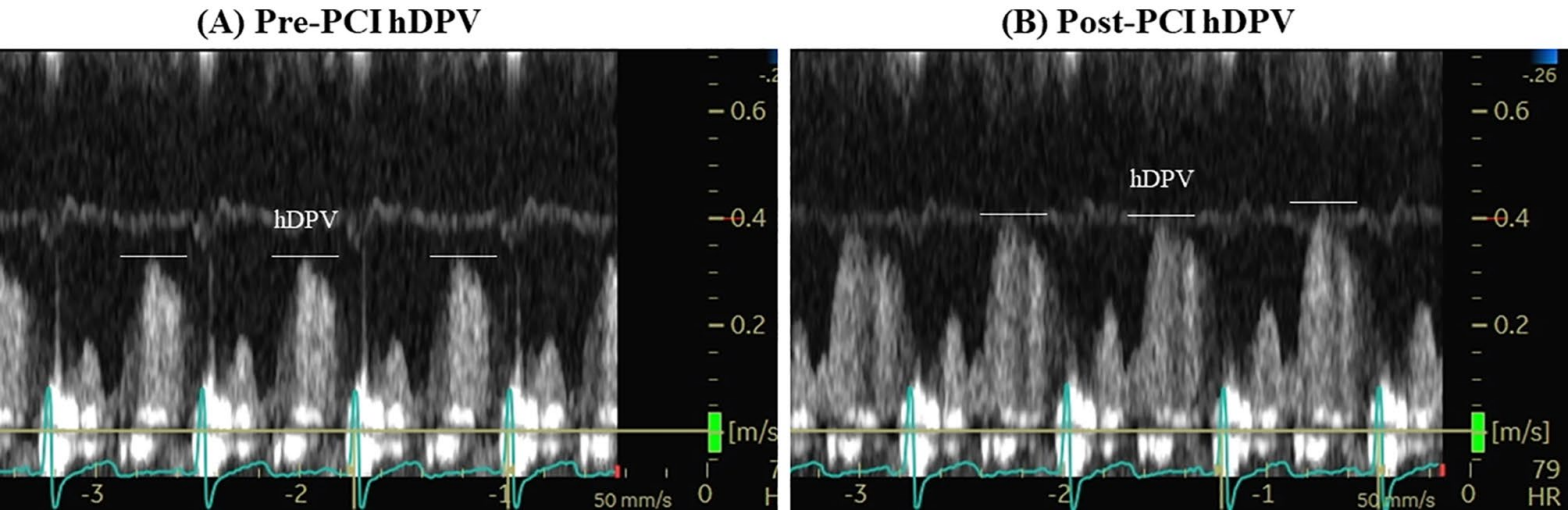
Representative images of coronary flow velocity measurements by transthoracic Doppler echocardiography. A representative case of a LAD lesion that underwent TDE examinations before and after successful PCI. (A) Pre-PCI hDPV, (B) post-PCI hDPV. *LAD* left anterior descending artery, *TDE* transthoracic Doppler echocardiography, *PCI* percutaneous coronary flow intervention, *hDPV* hyperaemic diastolic peak velocity.
